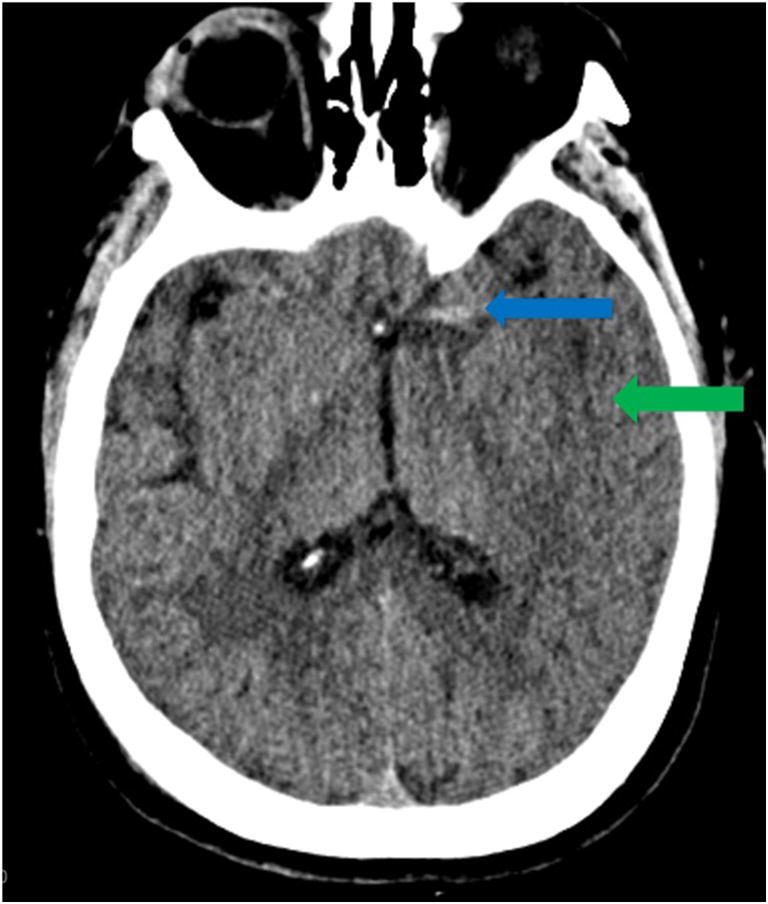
Acute thromboembolic infarction in a 57-year-old: Axial non-contrast CT scan demonstrates left hyperdense middle cerebral artery (MCA) sign (blue arrow), with increased density in the M1 segment of the left MCA, consistent with acute thromboembolic occlusion. There is subtle loss of gray-white matter differentiation, hypodensity, and engorgement along the left insular ribbon and temporal operculum (loss of insular ribbon sign), reflecting acute infarction (green arrow).
